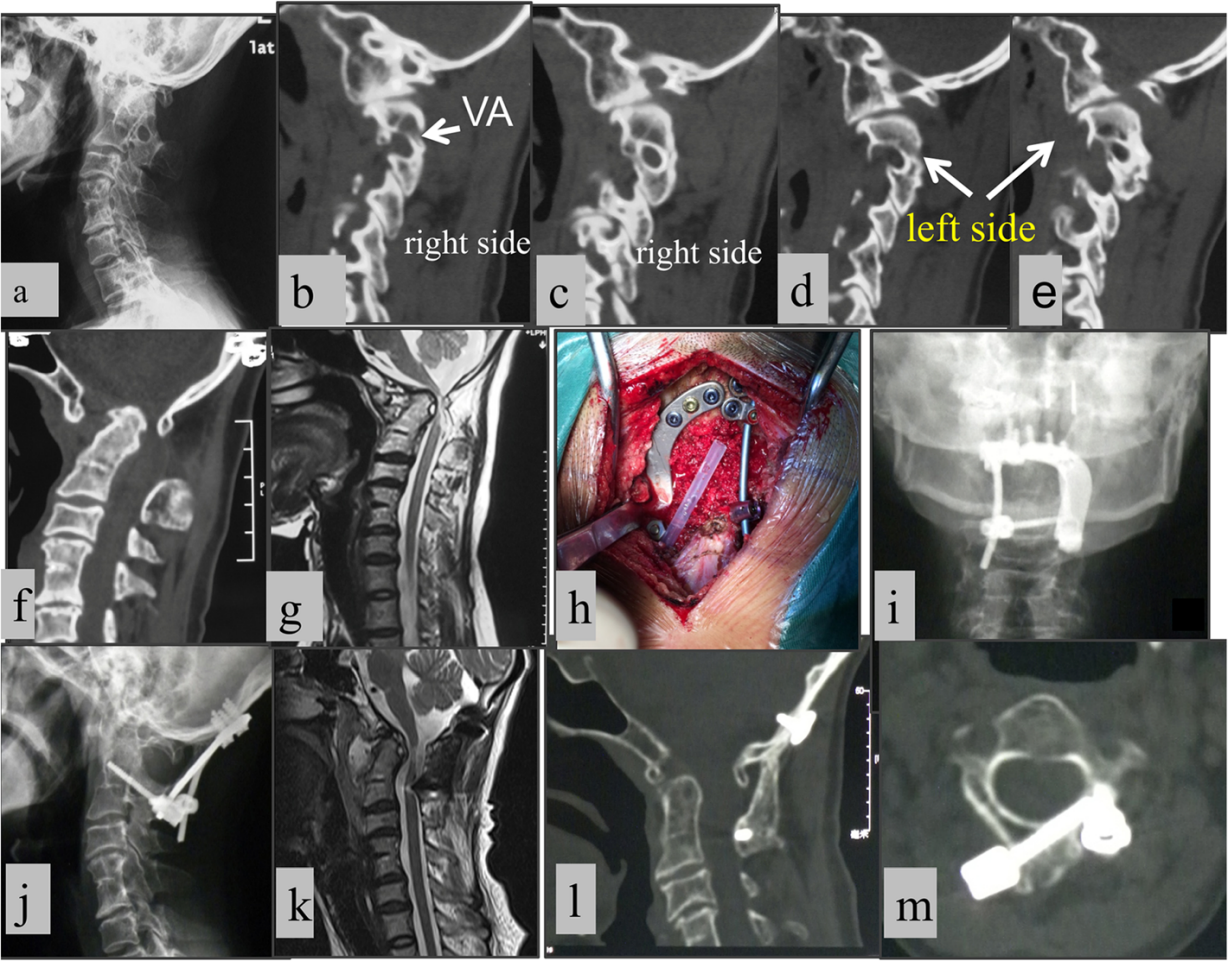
a The pre-operative radiographs of a 44-year-old woman showed sandwich AAD, C1 occipitalization and C2–3 and C5–6 congenital fusion. b-d Preoperative CT revealed the left C2 pedicle can accommodate a 3.5-mm screw (c), while the right pedicle was “thin”and occupied by VA. e. The odontoid invaded the foramen magnum, and ADI was abnormal (10 mm). f. The pre-operative mid-sagittal CT scan. g. The pre-operative MRI showed severe compression of the spinal cord. h. During the surgery: On the left side, C2PS was plated and connected with the occiput by a plate; On the right side, C2LS was inserted and connected with the occiput screws by a rod. Morselizedcancellous grafts harvested from the posterior iliac crest were bridged between the occiput and C2 lamina. i. The post-operative radiographs (AP). j. The post-operative radiographs (Lateral). k. The post-operative MRI showed adequate decompression. l. Postoperative CT (after 4 months) confirmed complete reduction and rigid fusion between occiput and C2. m. The postoperative CT showed the techniques included C2PS and C2LS
